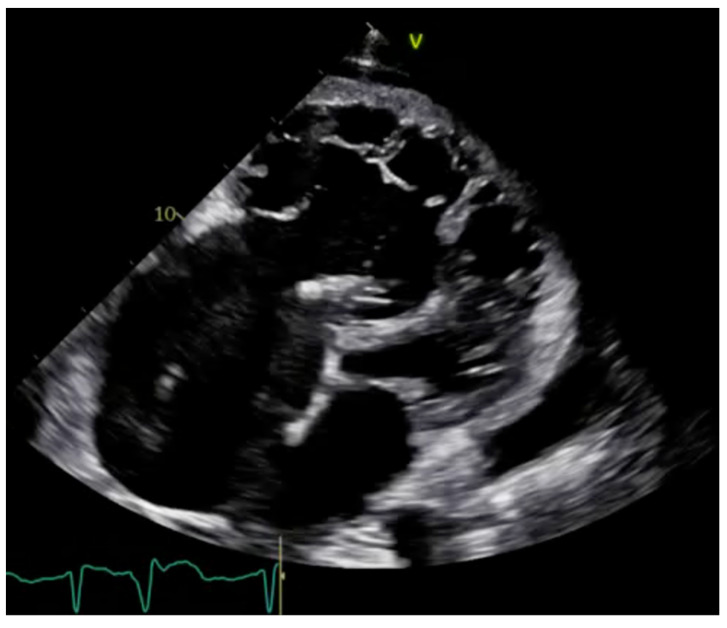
Arrhythmogenic right ventricular cardiomyopathy. Two-dimensional Transthoracic echocardiogram.
